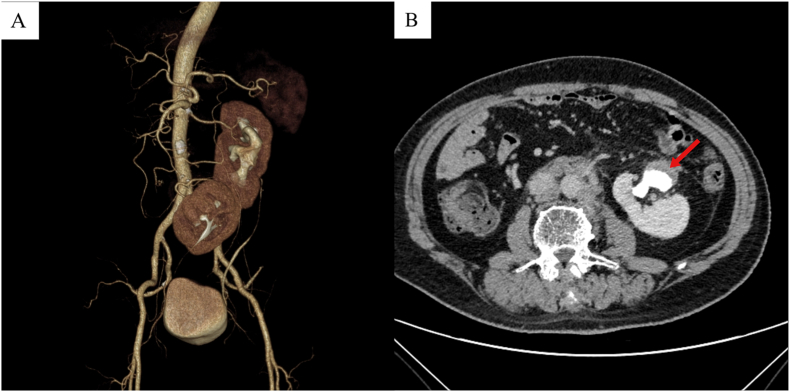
3D CT urography (CTU) imaging demonstrates the right kidney has crossed the midline, becoming inferiorly displaced and fused with the left kidney (A). Excretory phase CTU reveals a filling defect and a soft tissue density shadow in the left pelvis (B).
